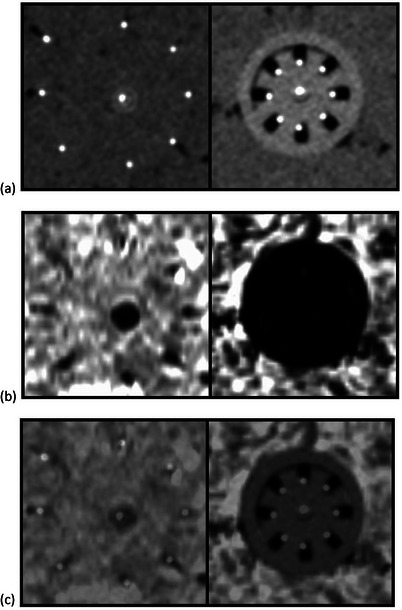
CT/MR image registration. Shown here are cross sections focusing on either the interstitial needles or the UIC body after CT/MR image registration. Panel (a) shows the CT image at a slice showing either the needles in tissue (left figure) or the applicator body (right figure), (b) shows the MR image at the same level (tissue in left and applicator body in right) after CT/MR registration, and (c) shows a 50/50 blend between the CT and MR images. UIC, universal interstitial cylinder.
